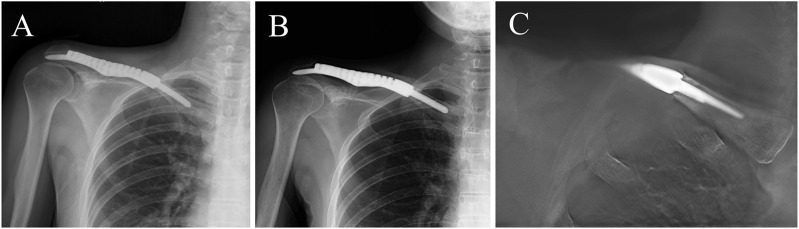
(A, B) 12-month and 36-month postoperative anteroposterior view X-ray. (C) The anteroposterior digital tomosynthesis graph showed no radiolucent lines around the intramedullary stem.
